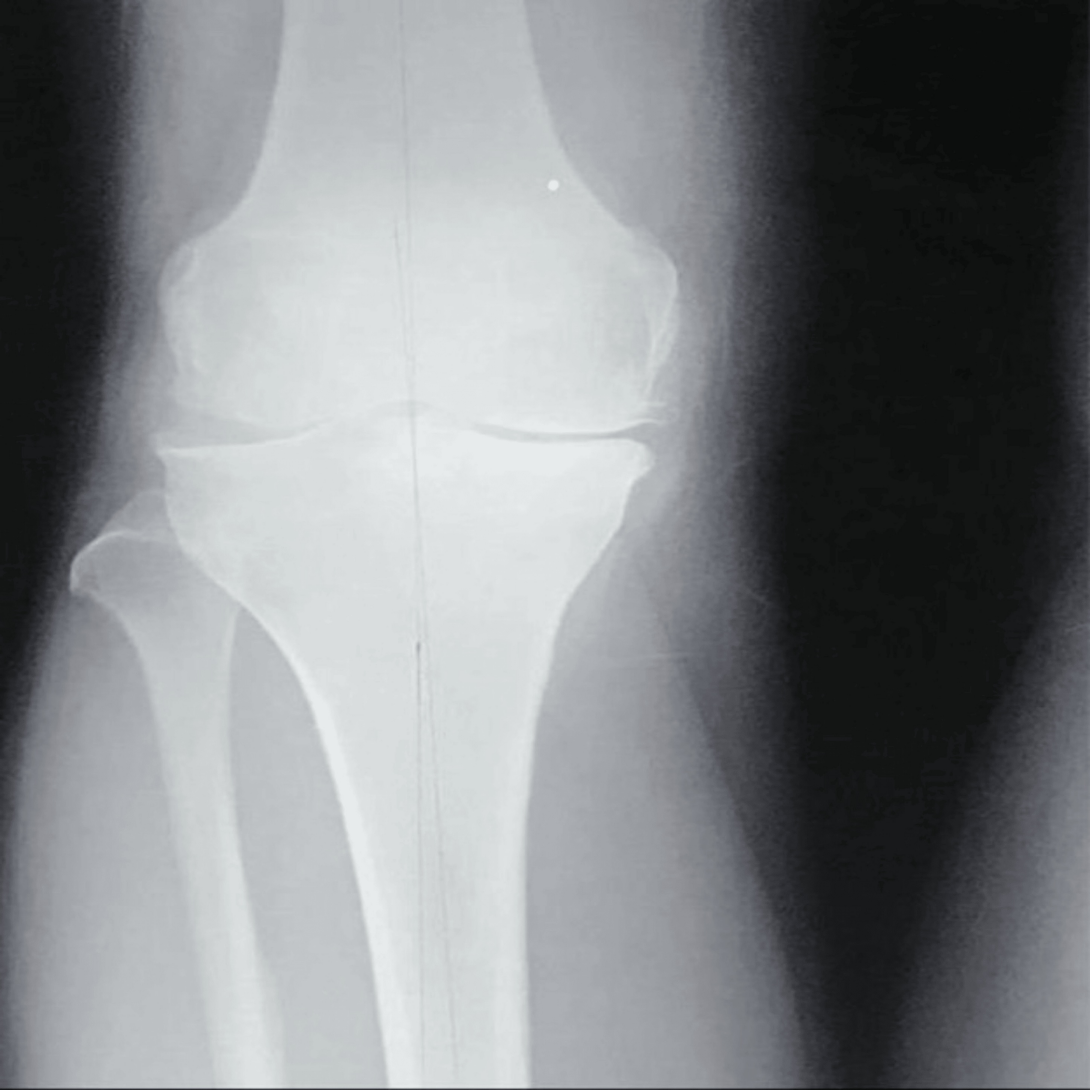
Preoperative anteroposterior radiograph demonstrating medial compartment osteoarthritis with medial joint space narrowing
